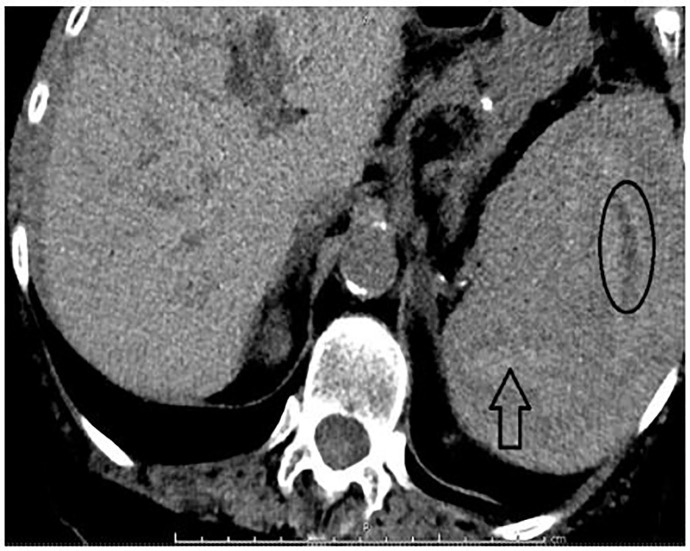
Computed tomography of abdomen and pelvis in transverse view showing multiple hypoechoic densities within splenic parenchyma suggestive of splenic laceration (arrow) and hematoma formation (oval).
